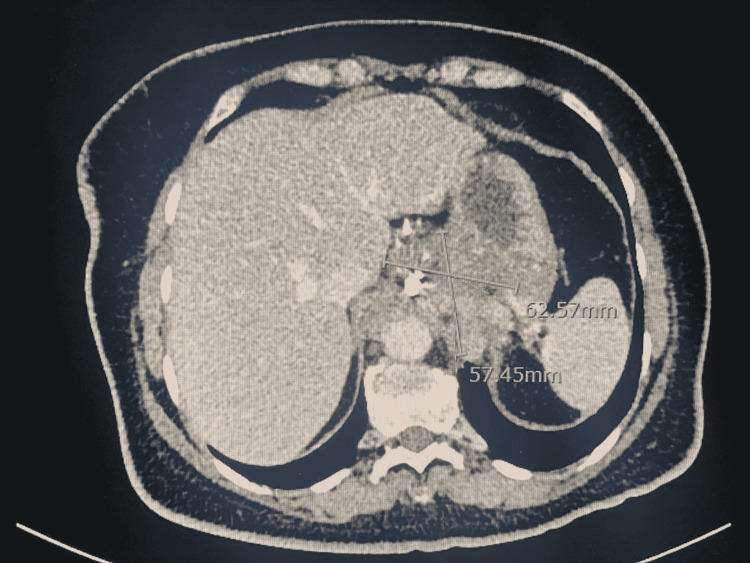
Computed tomography image showing the hemangioma after the coil embolization therapy
